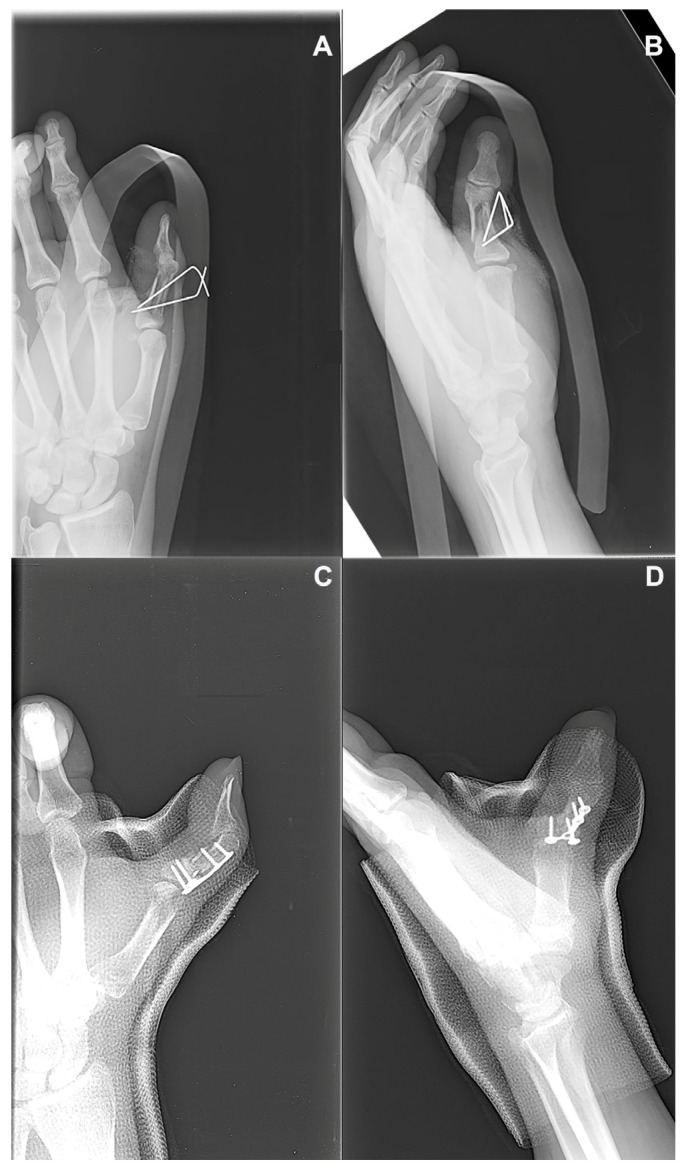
Radiographic images of the third metacarpal fracture site: (A,B) Preoperative anteroposterior and oblique views showing an atrophic nonunion characterized by the absence of bridging callus and sclerotic fracture margins, four months after initial fixation. (C,D) Postoperative radiographs obtained one month after the regenerative procedure, demonstrating progressive graft integration and early signs of fracture healing.
